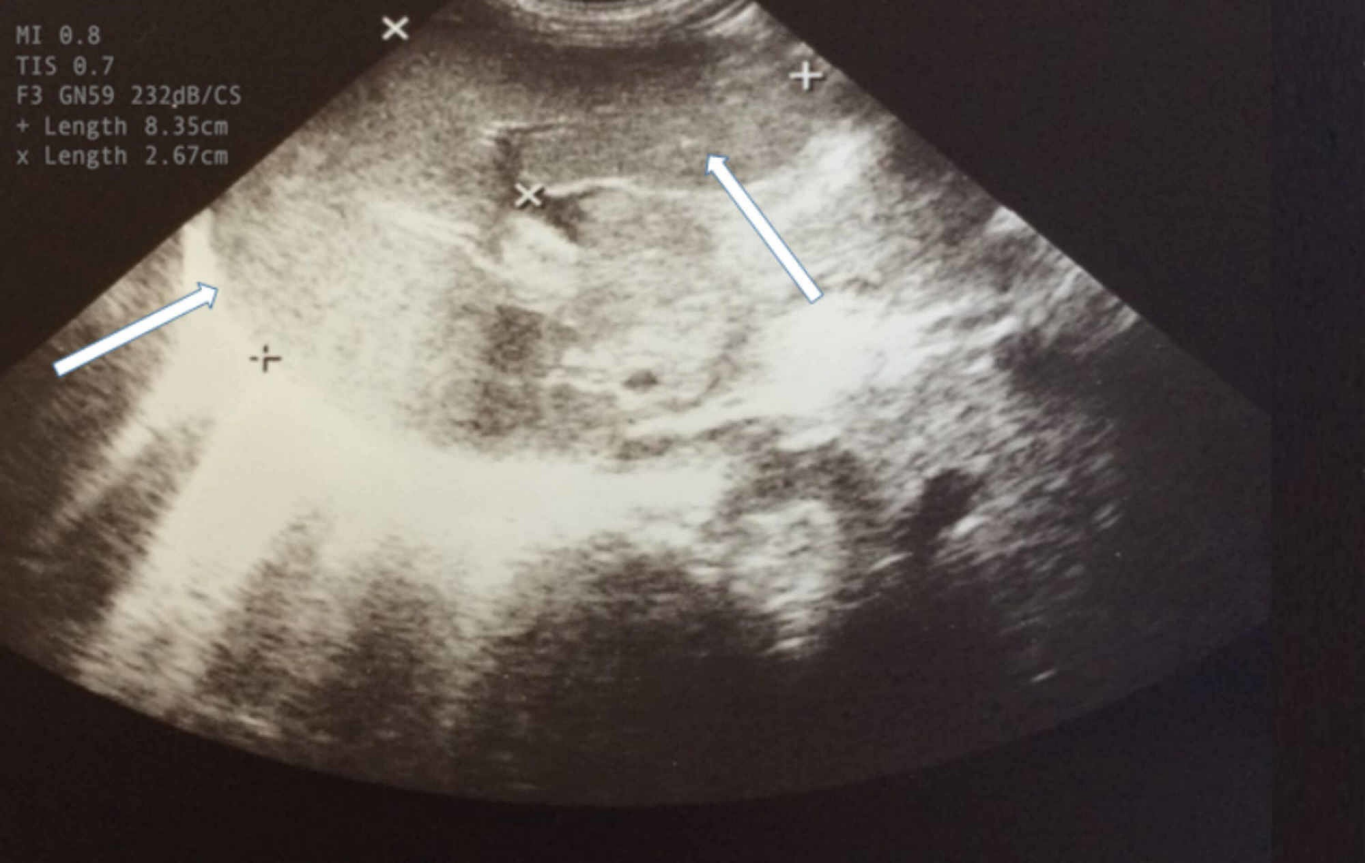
Patient's ultrasound. The second (repetitive) ultrasound showed splenomegaly (8.35 cm)
